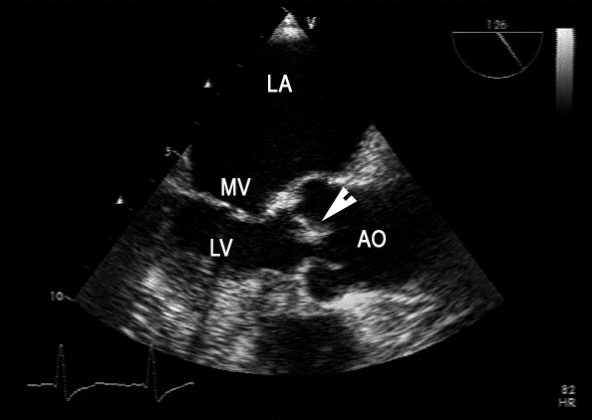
A transesophageal echocardiogram shows the left atrium (LA), parts of the mitral valve (MV), the left ventricle (LV), the ascending aorta (AO), and a thickened aortic cusp (arrow), the last of which is highly suggestive of endocarditis.
